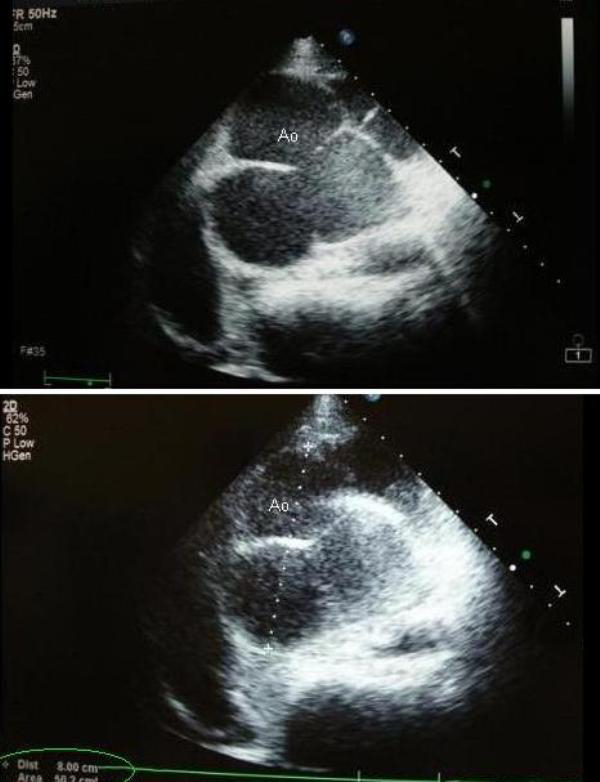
Echocardiography image of aorta. (A) Transthoracic echocardiography image of aorta, on parasternal short axis view, shows a dilated aorta at the level of aortic valves, measuring 80 mm (B).
